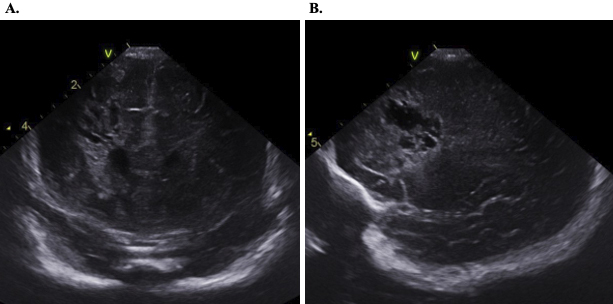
Intracranial ultrasonography. Ultrasonography on day 41 showed a cystic change with a surrounding hyperechoic around the right frontal horn of the lateral ventricle. Coronal (A) and sagittal views (B) are shown.
